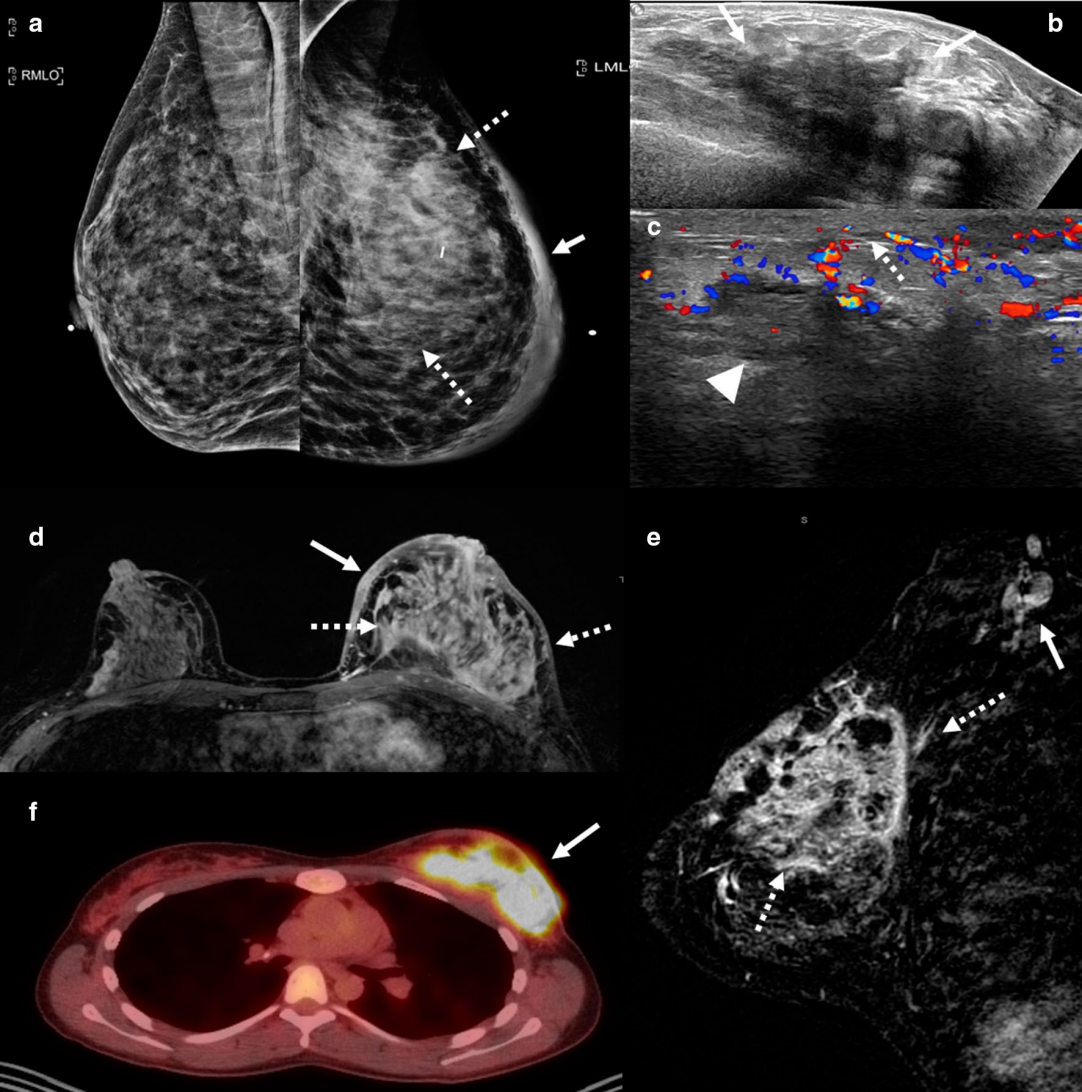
Granulomatous mastitis. A 34-year-old woman who is 2 years post-partum presented with a palpable left breast mass, diffuse breast swelling, tenderness, and erythema for several weeks. Bilateral mediolateral oblique mammogram (a) show diffuse skin thickening (solid arrow), global asymmetry, and trabecular thickening (dashed arrows) in the left breast, asymmetric from the right breast. Extended field of view grayscale ultrasound (b) shows an ill-defined hypoechoic, irregular mass (arrows). Color Doppler ultrasound (c) shows increased vascularity, edema (arrow head), and skin thickening (dashed arrow). T1weighted axial delayed post-contrast (d) and sagittal subtraction (e) post-contrast MRI shows diffuse skin thickening (solid arrow) and trabecular thickening with heterogenous enhancement involving the left superior breast (dashed arrows). There is associated axillary adenopathy (solid arrow in e). Axial PET/CT (f) shows diffuse fluorodeoxyglucose avidity involving the left breast (arrow). Findings and clinical presentation were suspicious for inflammatory breast cancer. The patient underwent three core needle breast biopsies of the mass in the left breast over the span of a month, and a skin punch biopsy. All biopsies showed dense stromal fibrosis, chronic inflammation, and features suggestive of granulomatous mastitis without atypia or malignancy
